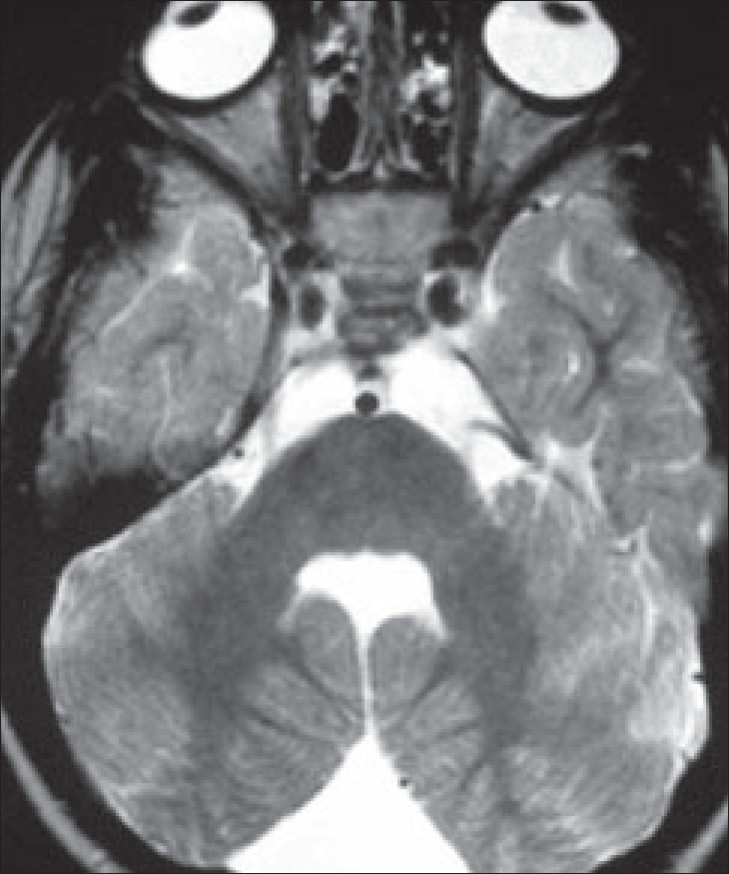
Axial T2W MRI of the brain
